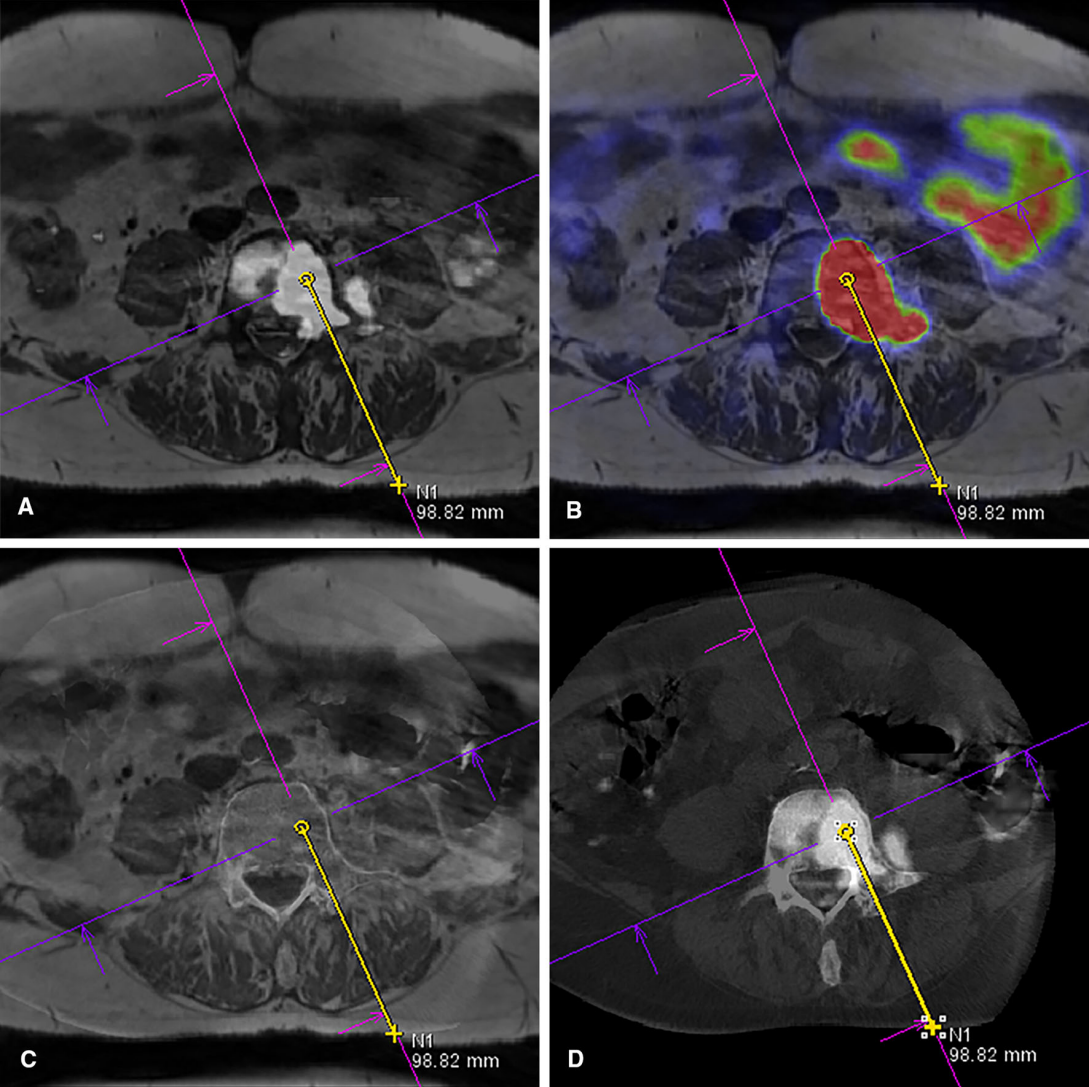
A–D Target planning of patient 1 on fused datasets. A The target was initially planned on a fusion of T1 and b800 images. B This target was then verified on a fusion of T1 and ^68^Ga-PSMA-PET images. C The CBCT scan showing the current anatomy was fused with the T1 to optimize the needle path. D The CBCT/b800 fusion with needle path was displayed on screens in the room during needle guidance
